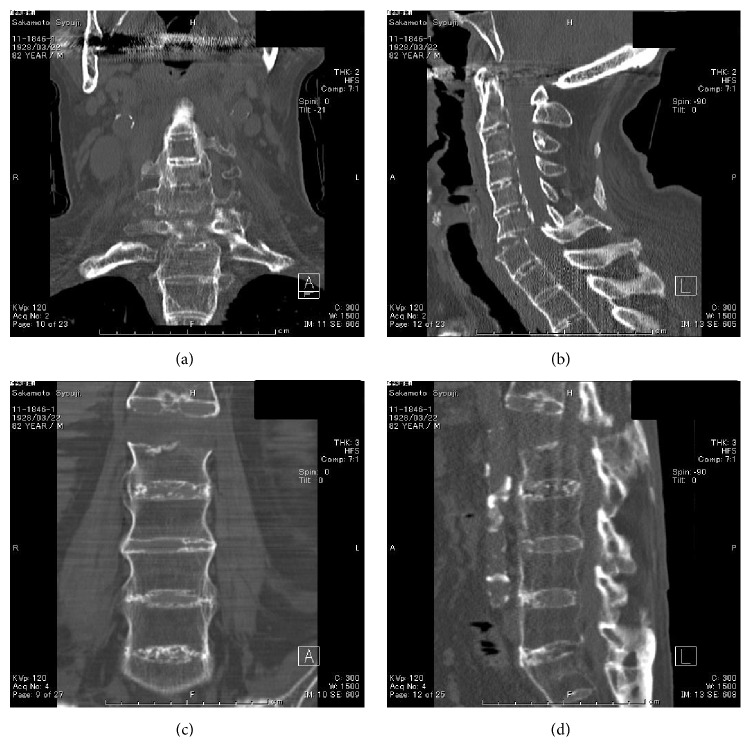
CT scan shows simultaneous cervical and lumbar spine fractures and dislocations at the level of C6-C7 ((a) and (b)) and L1 ((c) and (d)).
